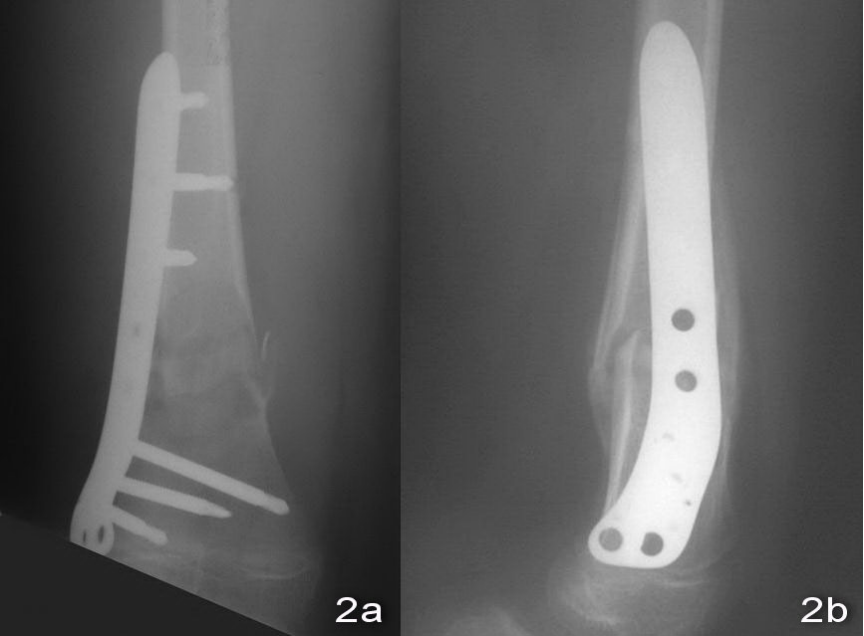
Post operative X-ray of right knee showed good alignment.
